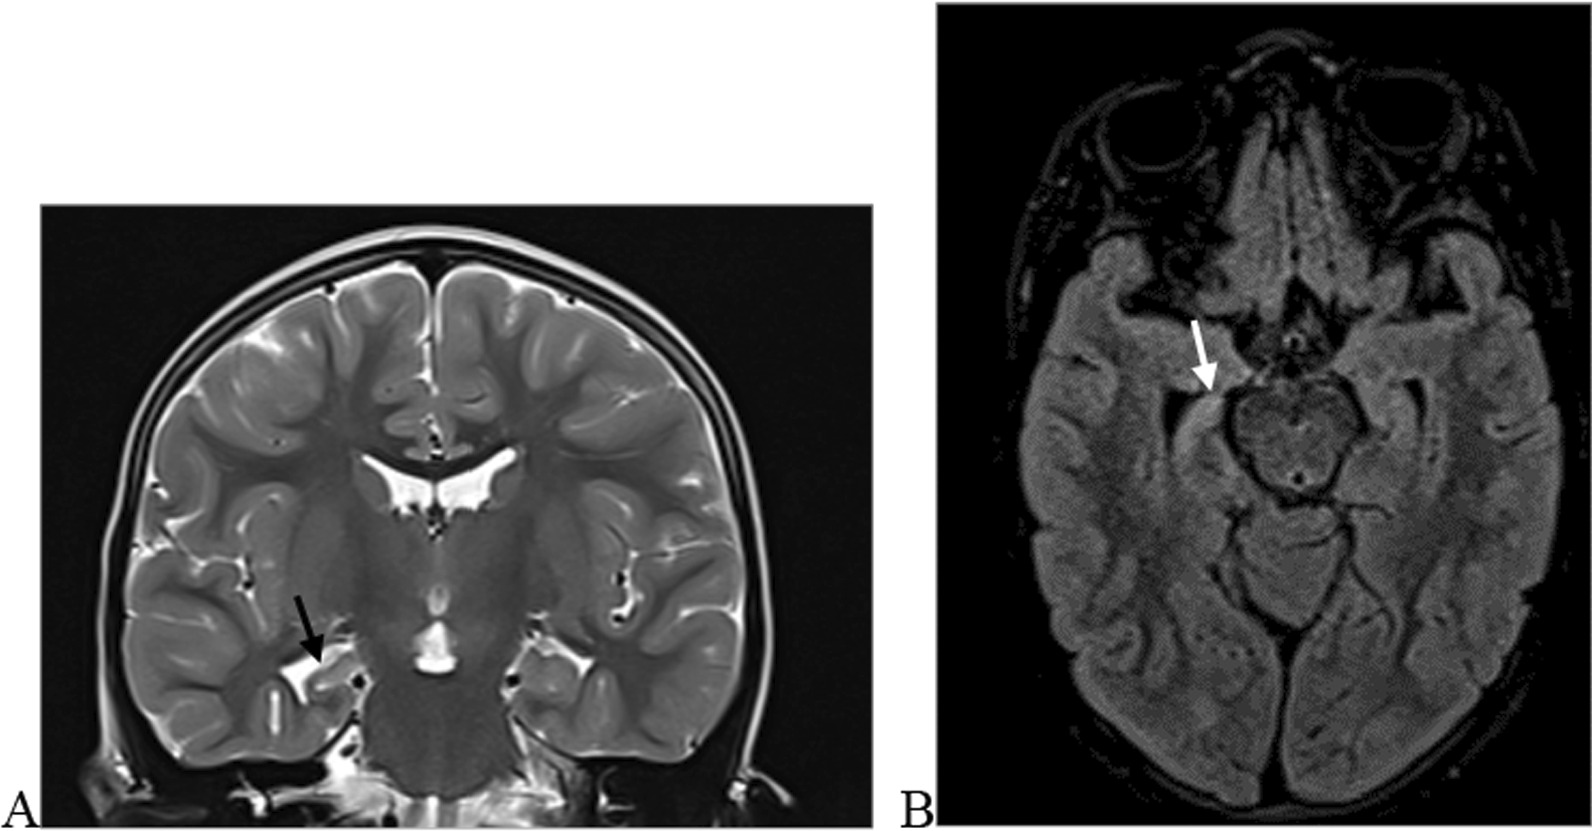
Right hippocampal sclerosis in a four-year-old male with focal seizures. Coronal T2 weighted image (WI) (A) and axial T2 FLAIR WI (B) images demonstrating an atrophied right hippocampus with dilated ipsilateral choroidal fissure and temporal horn of the lateral ventricle (arrow) and increased signal intensity on FLAIR due to gliosis (arrow) when compared to the contralateral hippocampus
